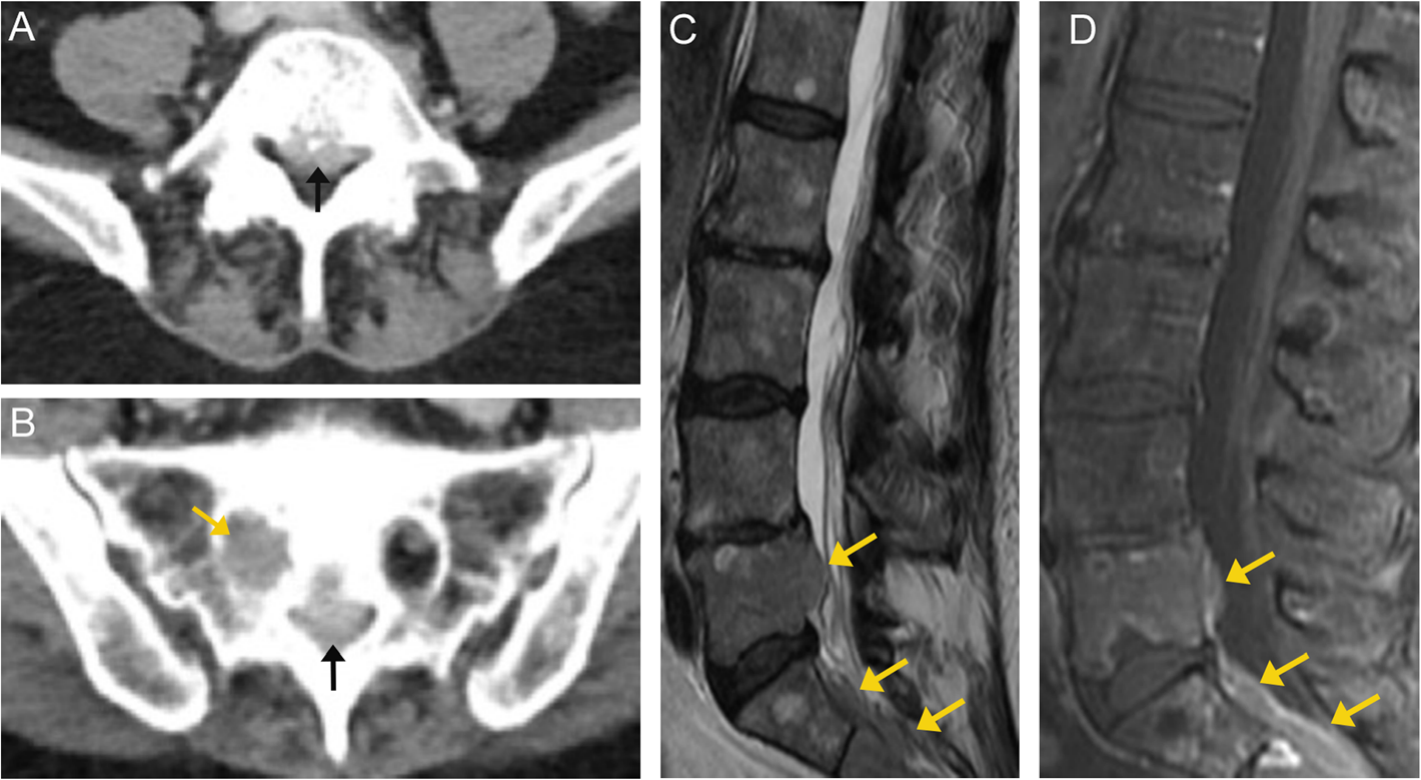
Lymphoma. Axial CT images through the lumbar spine (a) and sacrum (b) demonstrate abnormal soft tissue within the spinal canal (black arrows). Additionally, there is soft tissue replacement of the normal fat in the right sacral foramen (orange arrow). T2-weighted MR image (c) of the lumbosacral spine demonstrates an intermediate to low signal lesion involving the posterior aspect of L5, and another component at S1-S2 (orange arrows). There is no involvement of the intervertebral discs. Post-contrast T1-weighted fat-suppressed image (d) demonstrates relatively homogeneous enhancement
